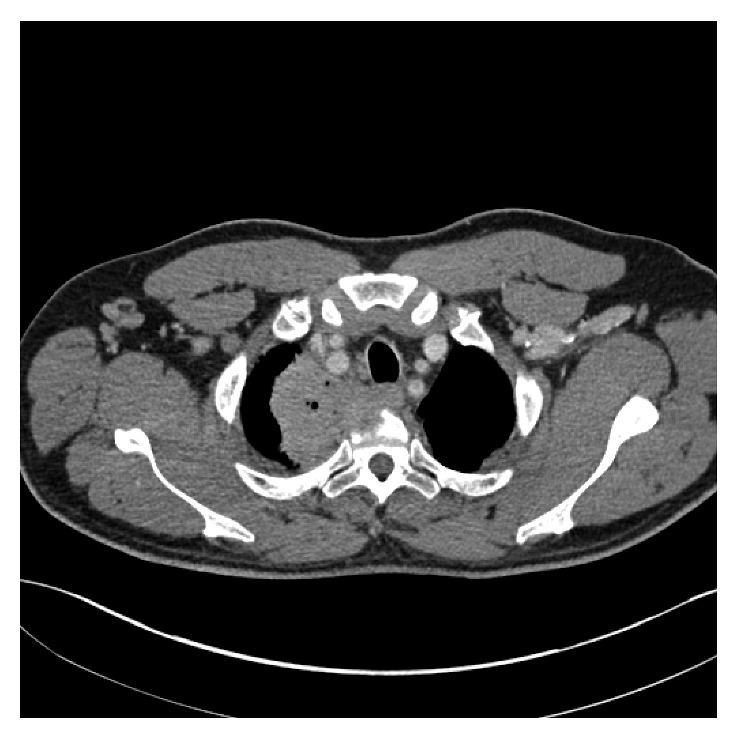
Computed tomography scan of the chest showing right superior sulcus mass invading a vertebral body.
